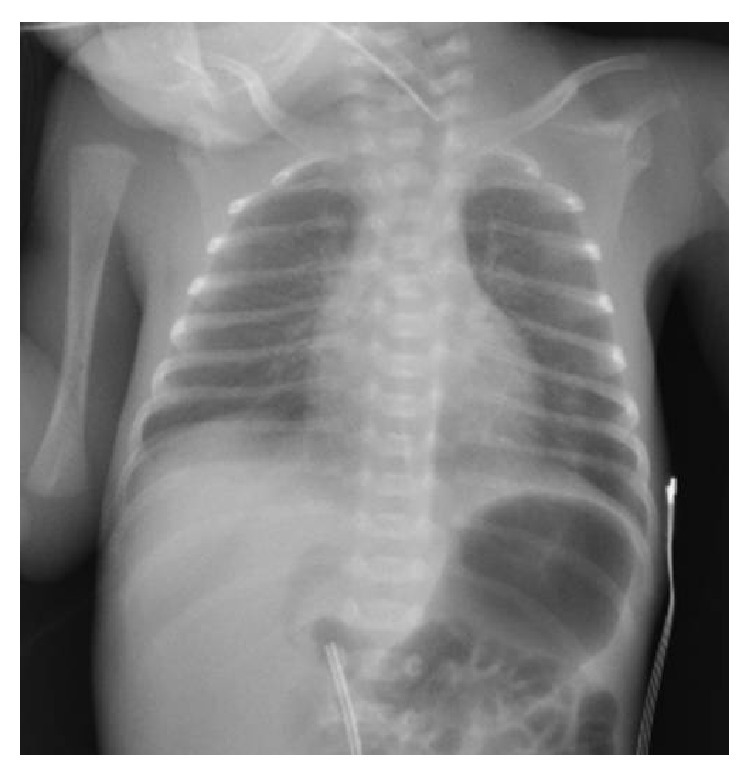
Chest radiograph (first day of life) revealing diffuse haziness of both lungs.
